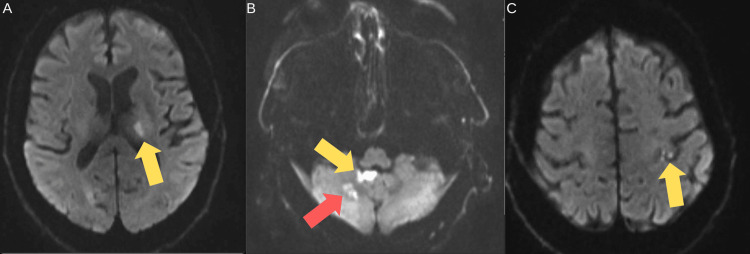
Magnetic resonance imaging of the head sequences showing recurrent infarcts Diffusion-weighted images show infarcts in the left thalamus (A), right cerebellar hemisphere (red arrow), vermis (yellow arrow) (B), and left frontal cortex (C).
